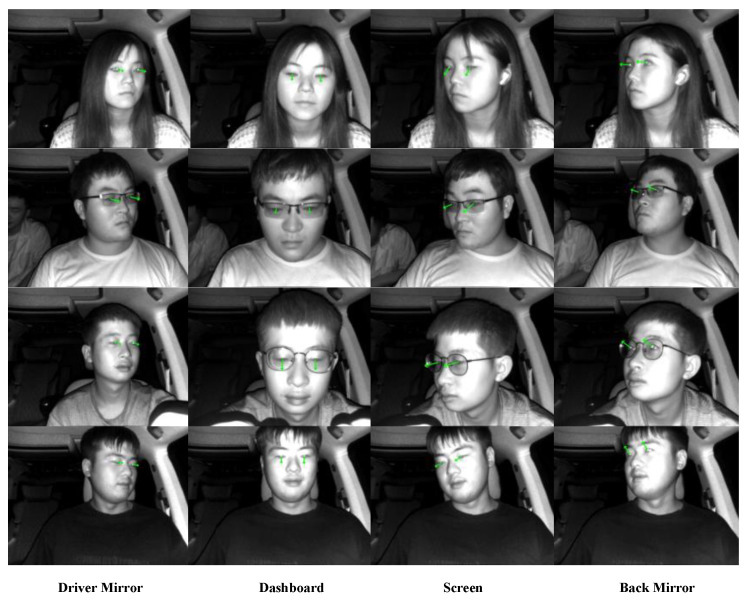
Detection results of driver’s partial gaze points in the interior of a Toyota business SUV. Green arrows indicate the driver’s gaze direction detected by our gaze-estimation model.
